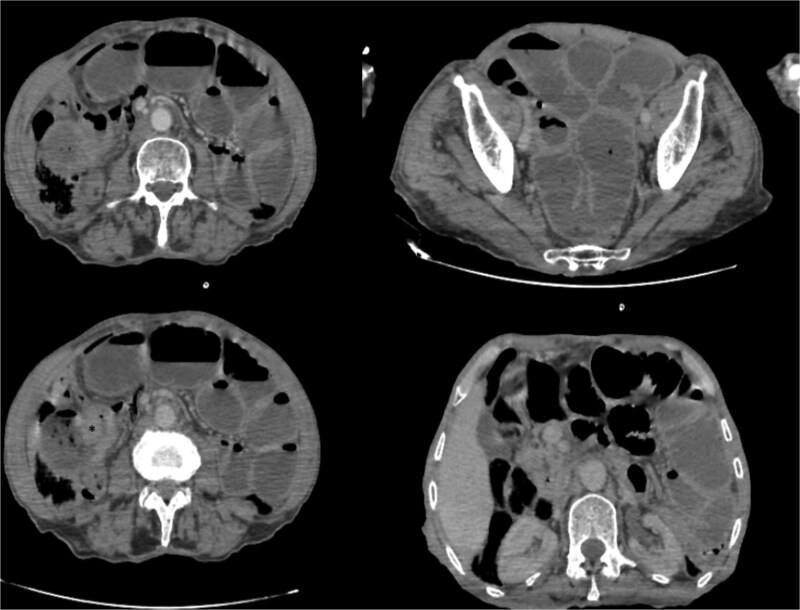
Abdominal computed tomography scan showing dilated jejunal loops; (*): Ileal mass.
